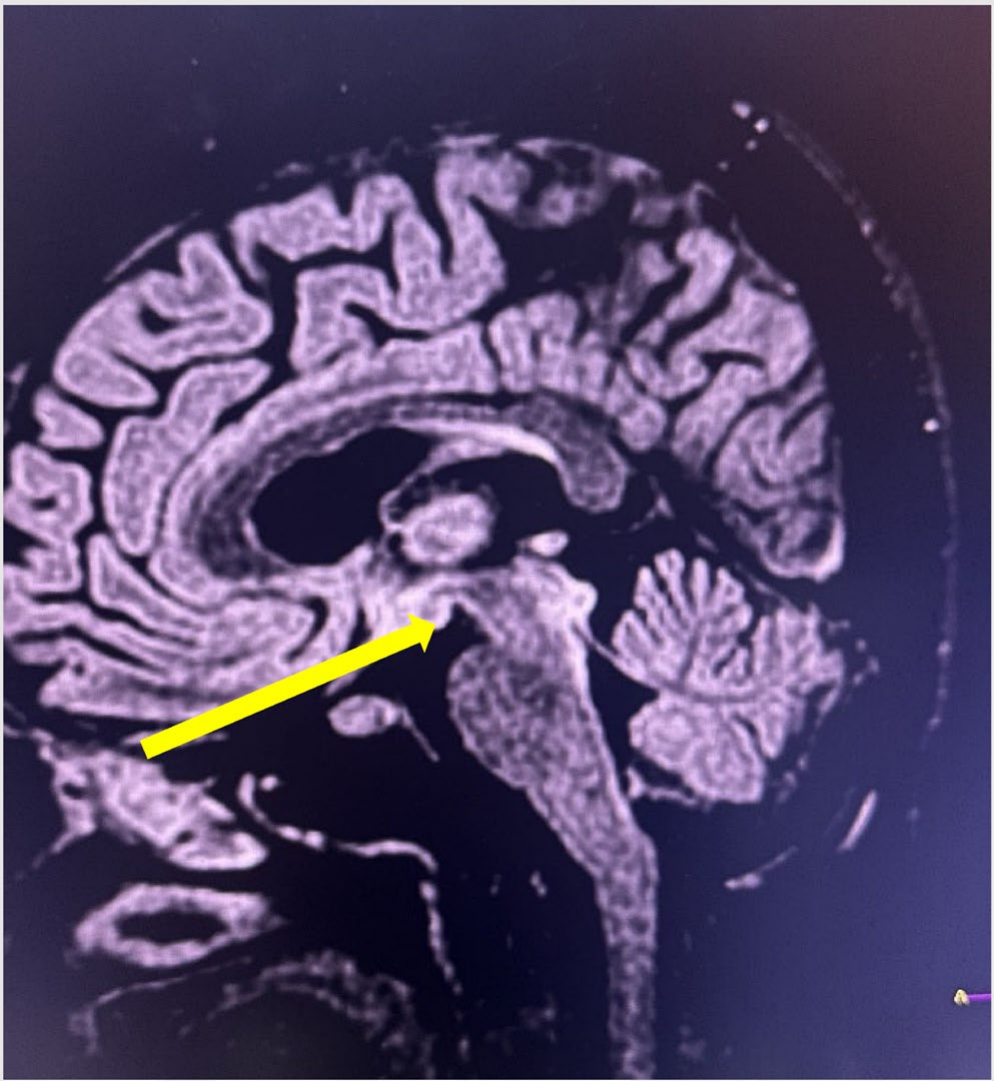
Cerebral MRI T2/FLAIR hyperintensity involving the mamillary bodies (yellow arrow), suggestive of WE.
